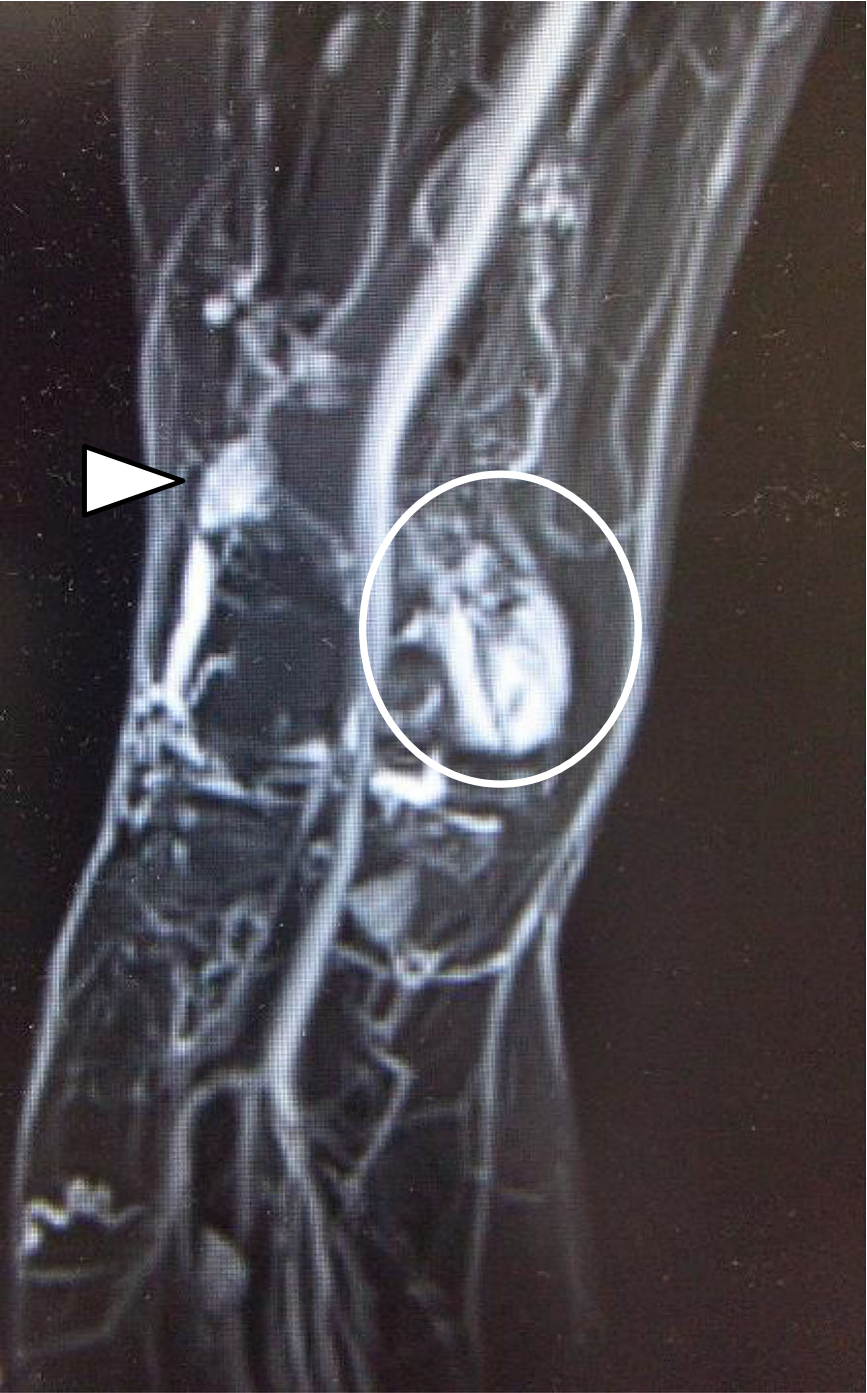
Magnetic resonance angiogram shows a hyperintense region (circle) on the medial side of the knee joint, consistent with the magnetic resonance image. Moreover, another hyperintense region is also indicated near the lateral femoral condyle (arrowhead) and was suspected of being a hemangioma
